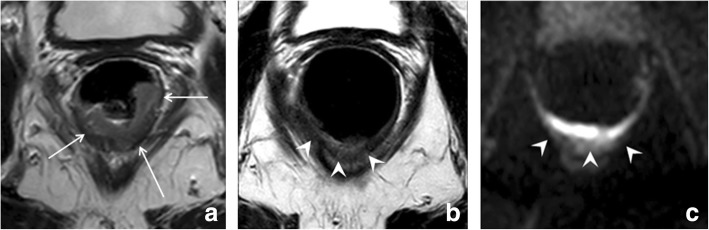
Susceptibility artifacts on DWI. Pre-treatment T2-weighted MRI (a), post-CRT T2-weighted (b), and post-CRT b1000 diffusion-weighted (c) images of a female patient treated for a low rectal tumor (arrows in a). On the post-CR images, a large amount of gas is present within the rectal lumen, which causes an artificial pile-up of signal projecting over the dorsal rectal wall at the site of the previous tumor bed (arrowheads in b and c). If not recognized as an artifact, this signal may be falsely interpreted as tumor
